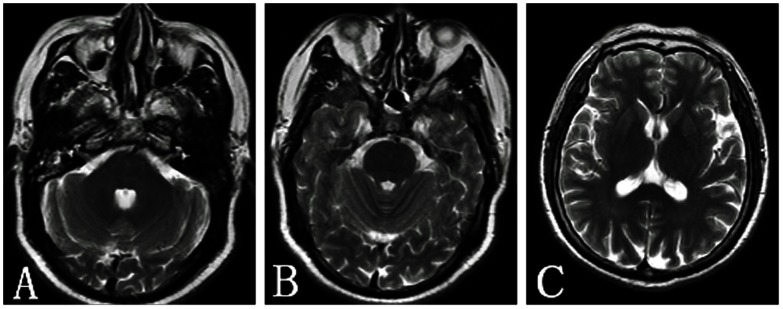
A 47-year-old man with acute otitis media with effusion of MRI abnormalities in the right middle ear, bilateral maxillary sinuses and sphenoid sinus. A: The right mastoid cavity and middle ear cavity show hyperintensity on T2WI. A–B: The bilateral maxillary sinuses and sphenoid sinus show hyperintensity on T2WI. C: No abnormality can be observed in the brain on T2WI.
